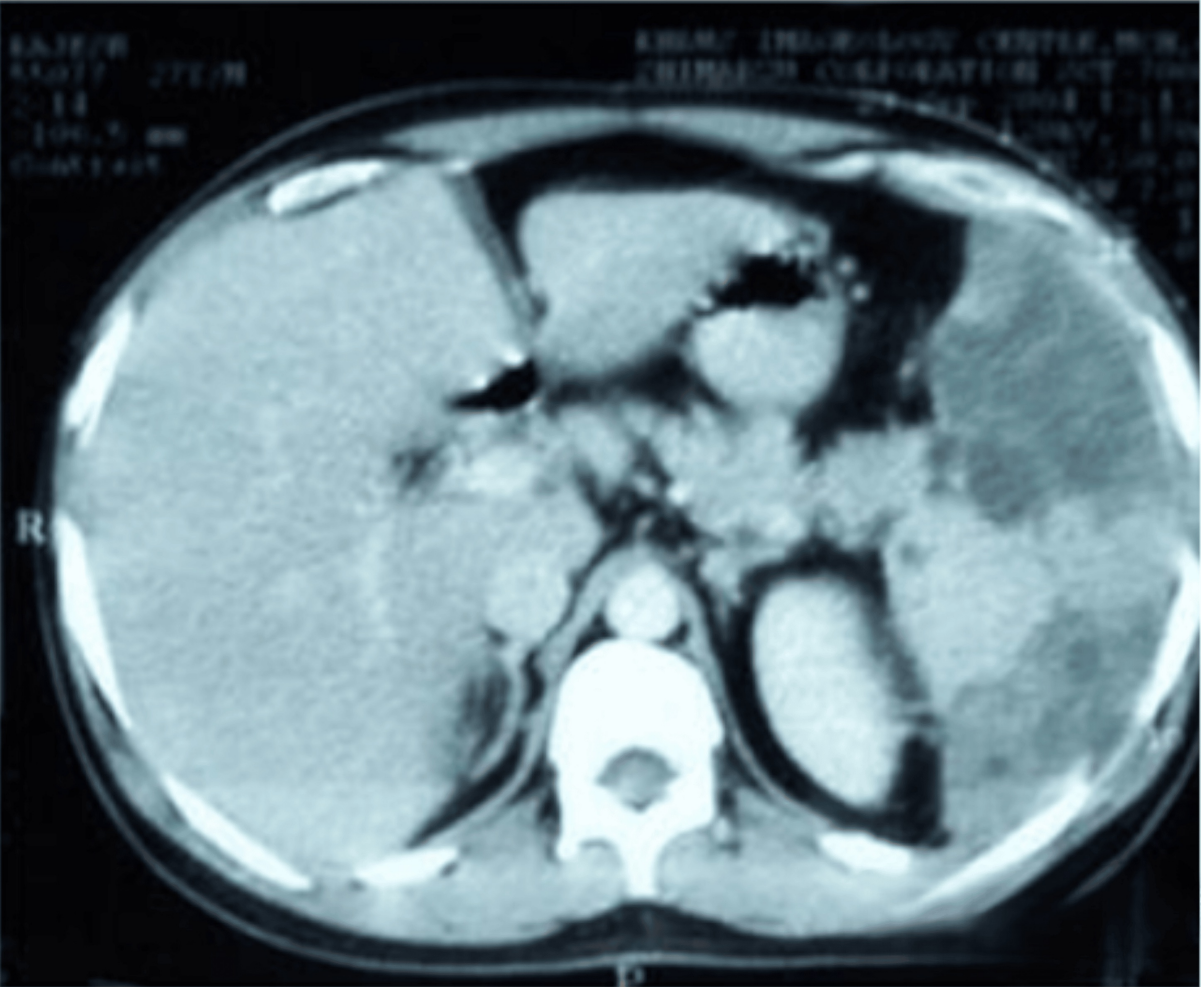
CT of the abdomen showing multiple hepatic and splenic abscesses. Axial contrast-enhanced CT scan of the abdomen demonstrating multiple, well-circumscribed hypodense lesions of varying sizes in both the liver and spleen, consistent with multiple visceral abscesses.
